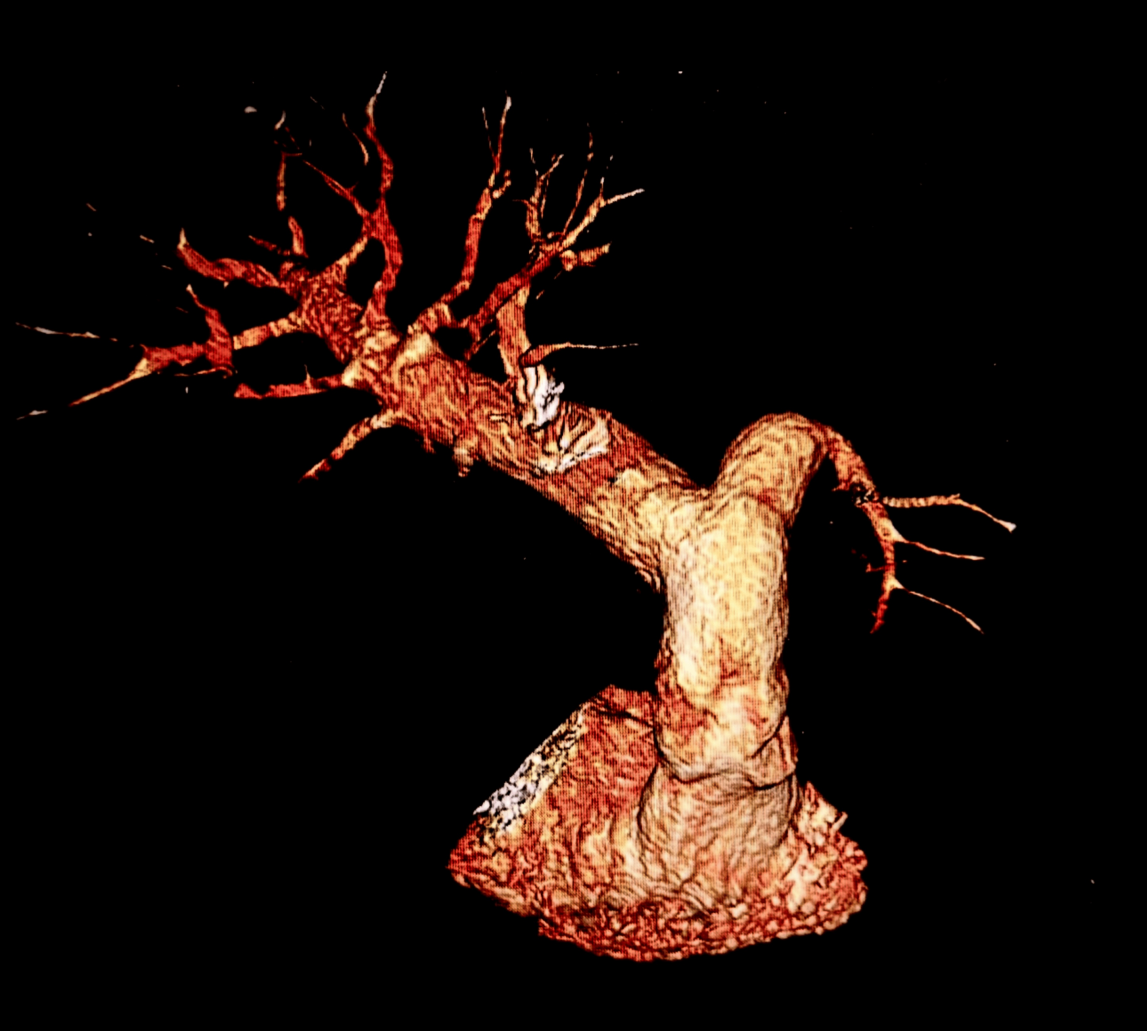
Three-dimensional computed tomography reconstruction of the pulmonary arteries Three-dimensional reconstruction from contrast-enhanced computed tomography showing the pulmonary arterial tree. Irregularity and narrowing of segmental branches, with areas of reduced distal perfusion, are observed - consistent with chronic thromboembolic pulmonary hypertension.
